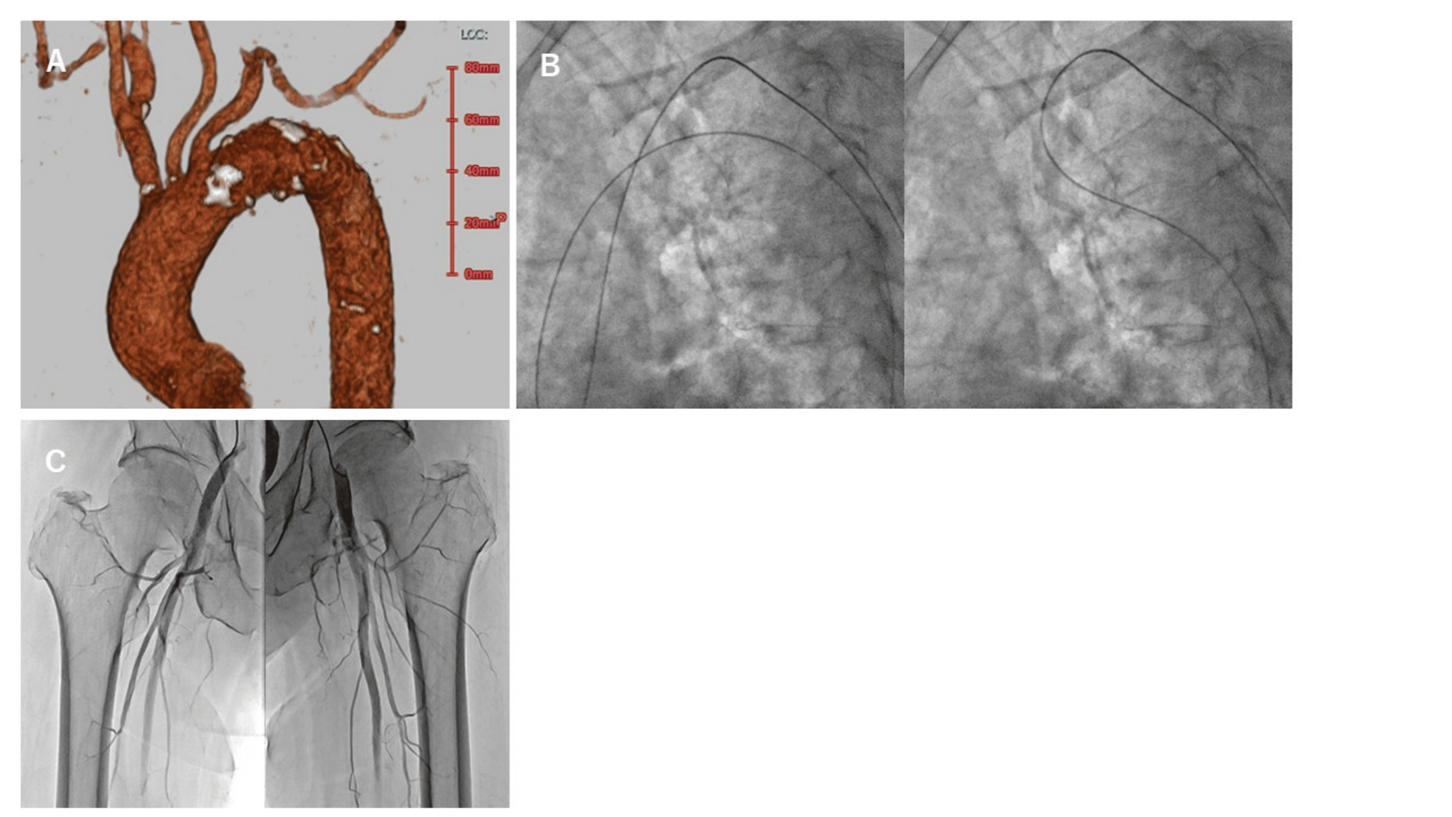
Lower limb angiography in Case 2. (A) The aortic arch is type III on contrast-enhanced computed tomography. (B) A 0.035-inch guidewire was advanced into the descending aorta while being turned around at the aortic valve (left figure). After the 4-French catheter was guided into the descending aorta, it was pulled up, the bend was removed, and it was extended (right figure). (C) The tip of the catheter was advanced to the common femoral artery on both sides.
